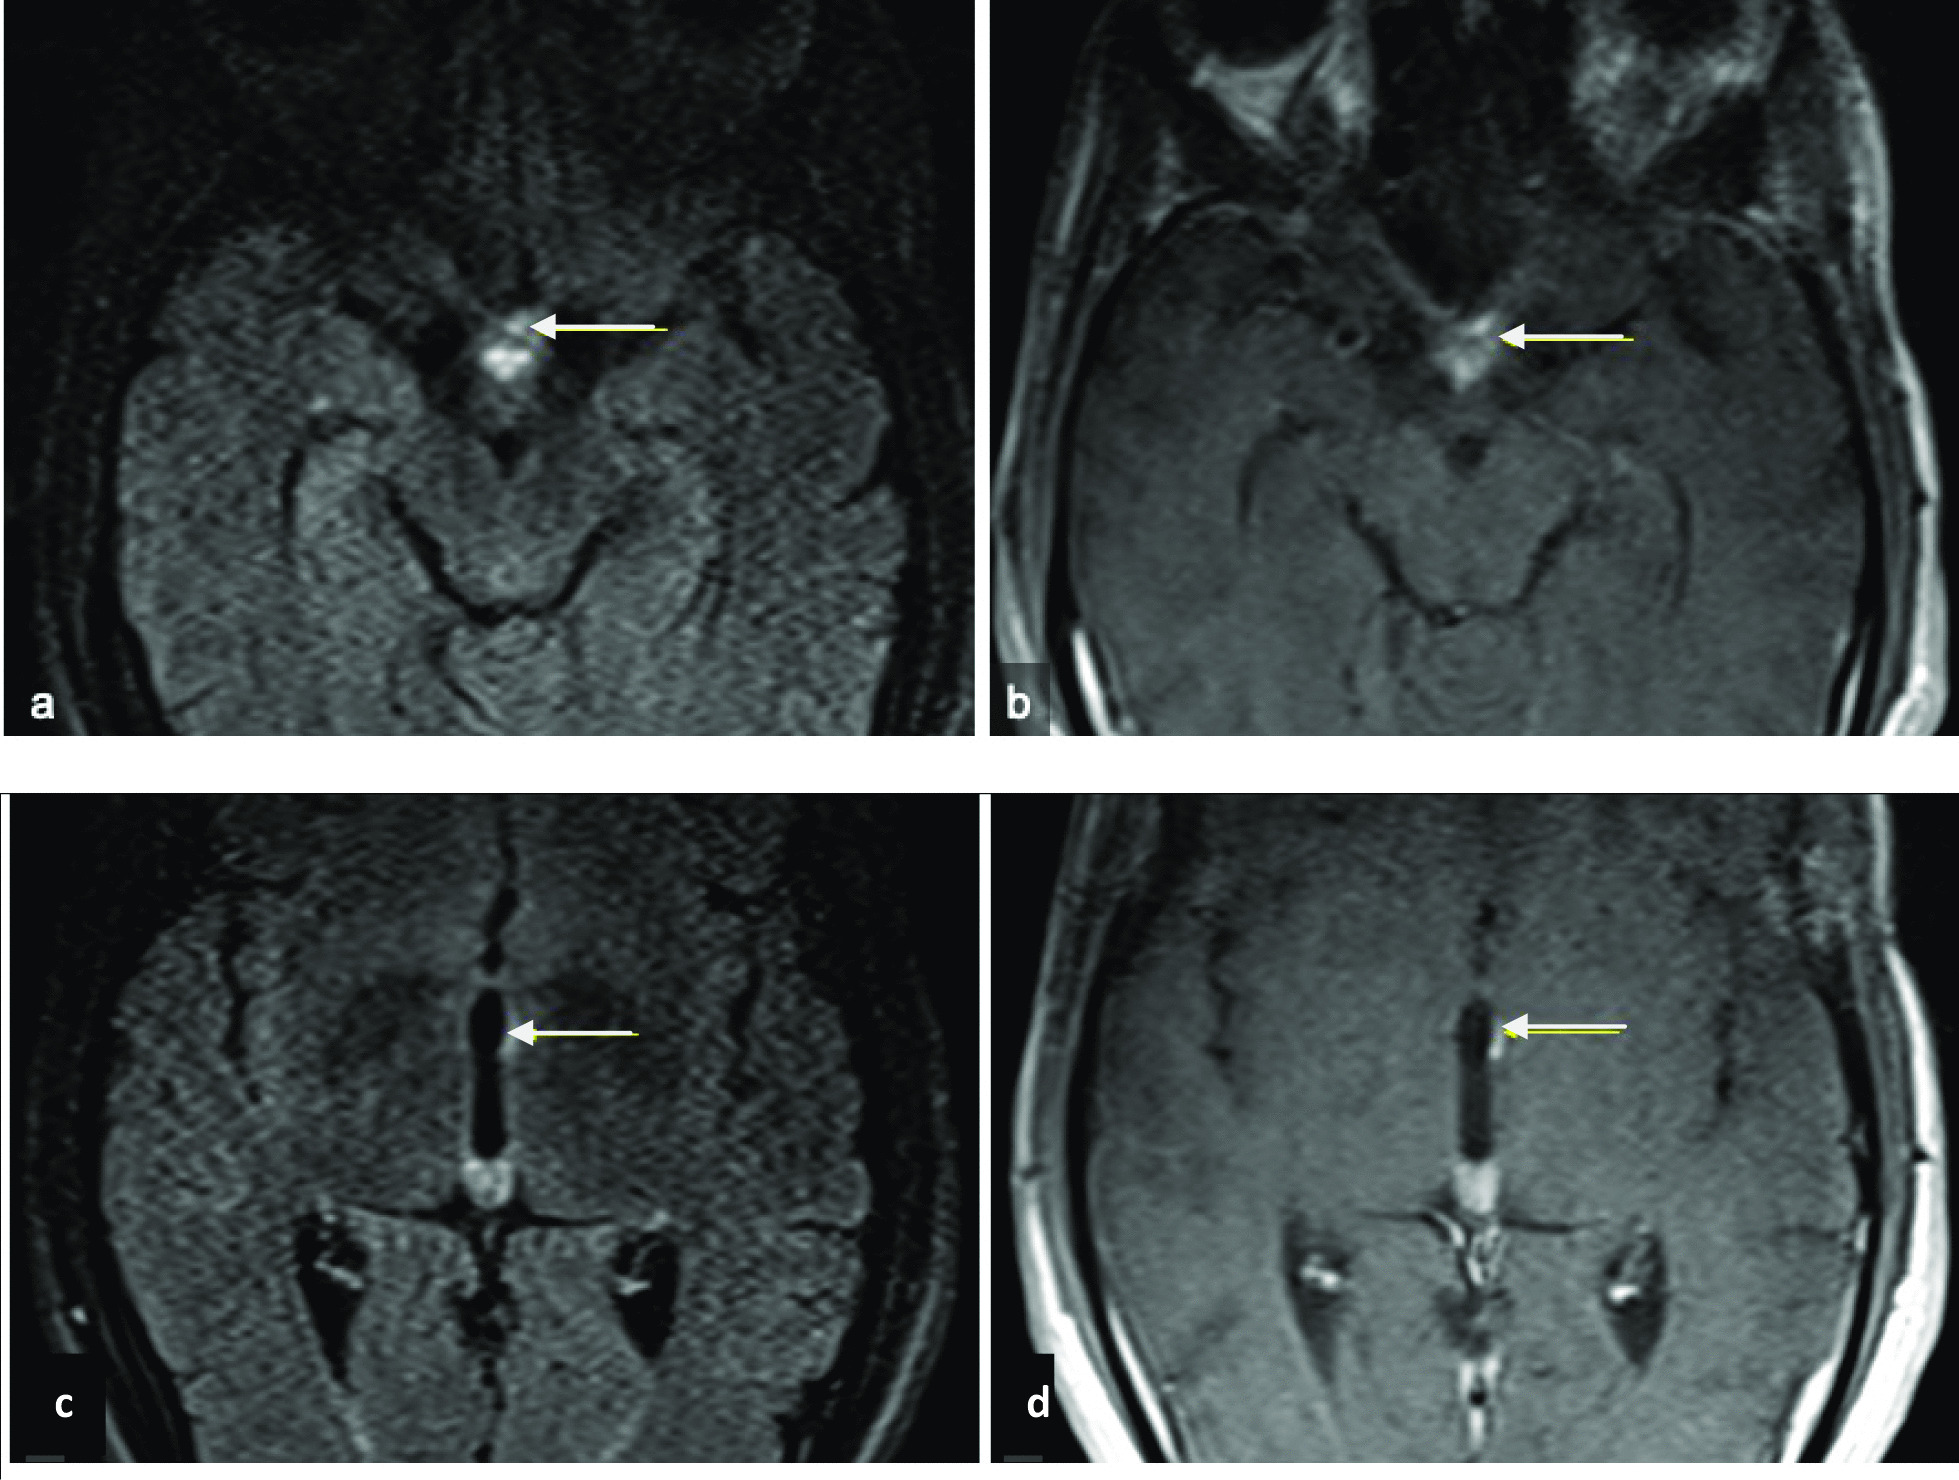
a–d Brain magnetic resonance images. a Axial FLAIR-weighted image showing hyperintensity of the optic chiasma (arrow); b axial T1 post-contrast administration weighted MRI image showing enhancement of the optic chiasma extending to the right optic nerve (arrow); c axial FLAIR-weighted image showing a focal hyperintensity of the left wall of the third ventricle (arrow); d axial T1 post-contrast administration weighted image showing enhancement of the left wall of the third ventricle (arrow)
